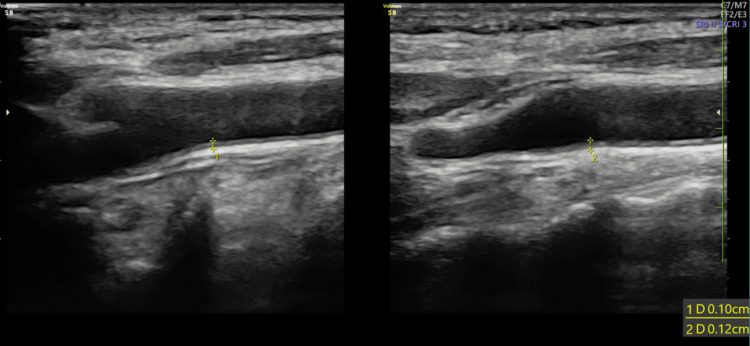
B-mode ultrasonography of bilateral common carotid artery (longitudinal view) shows carotid intima-media thickness of 1.0 and 1.2 mm in the far wall of right and left common carotid artery, respectively.
